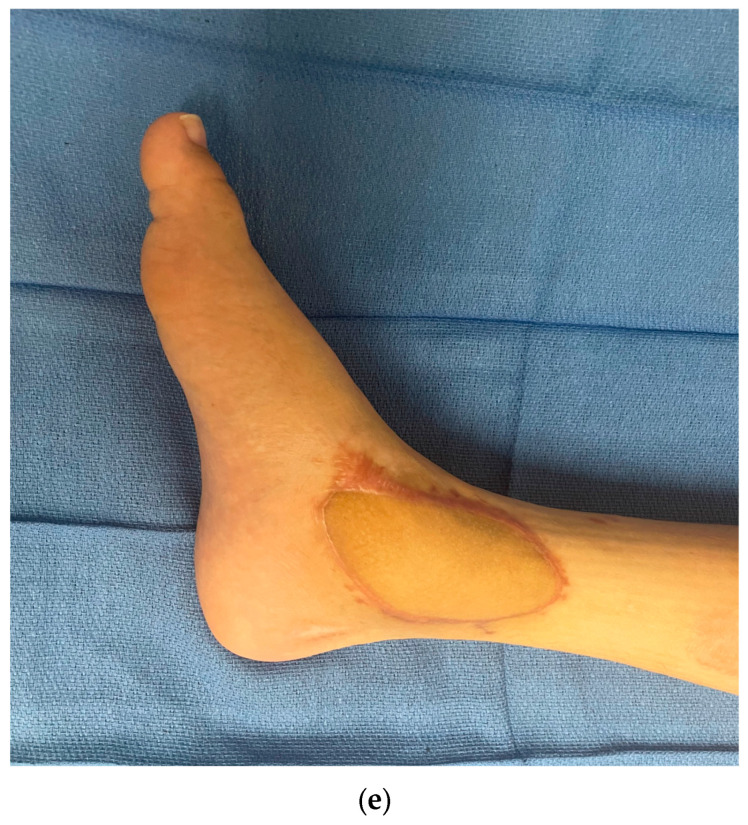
Color duplex ultrasonography facilitates planning of thin flaps through precise perforator location. (a) CDU mapping of thin SCIP flap demonstrating superficial branch (*short arrow*) and deep branch (*long arrow*). (b) Flap design based on CDU mapping. (c) Completion of flap elevation above level of superficial fascia. Postoperative photos (d) immediately and (e) 6 months after surgery. Patient of senior author A.A.S.
